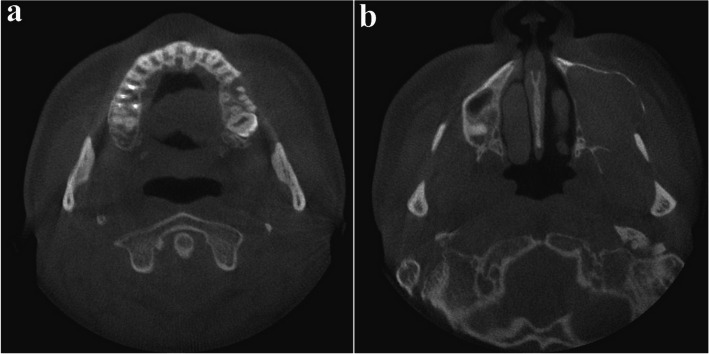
CT imaging, (a) showing radiolucent lesion involving left upper third molar, (b) showing large cystic lesion with buccal cortical expansion and perforation in left maxillary sinus
